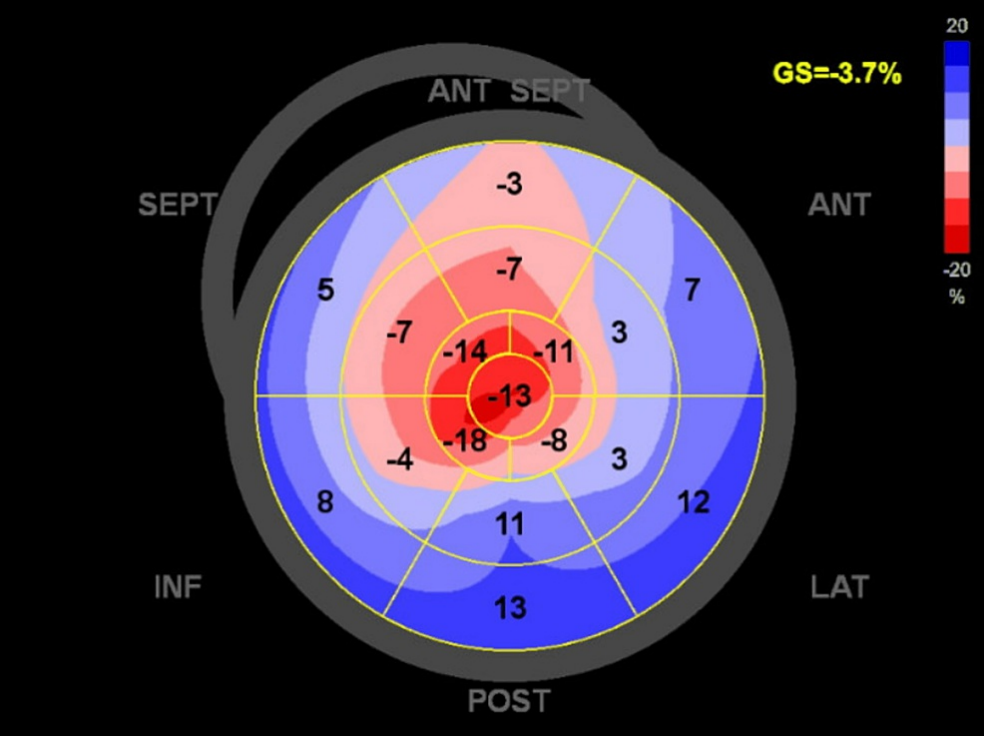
Longitudinal strain bull's eye plot pattern in echocardiogram showing apical sparing
